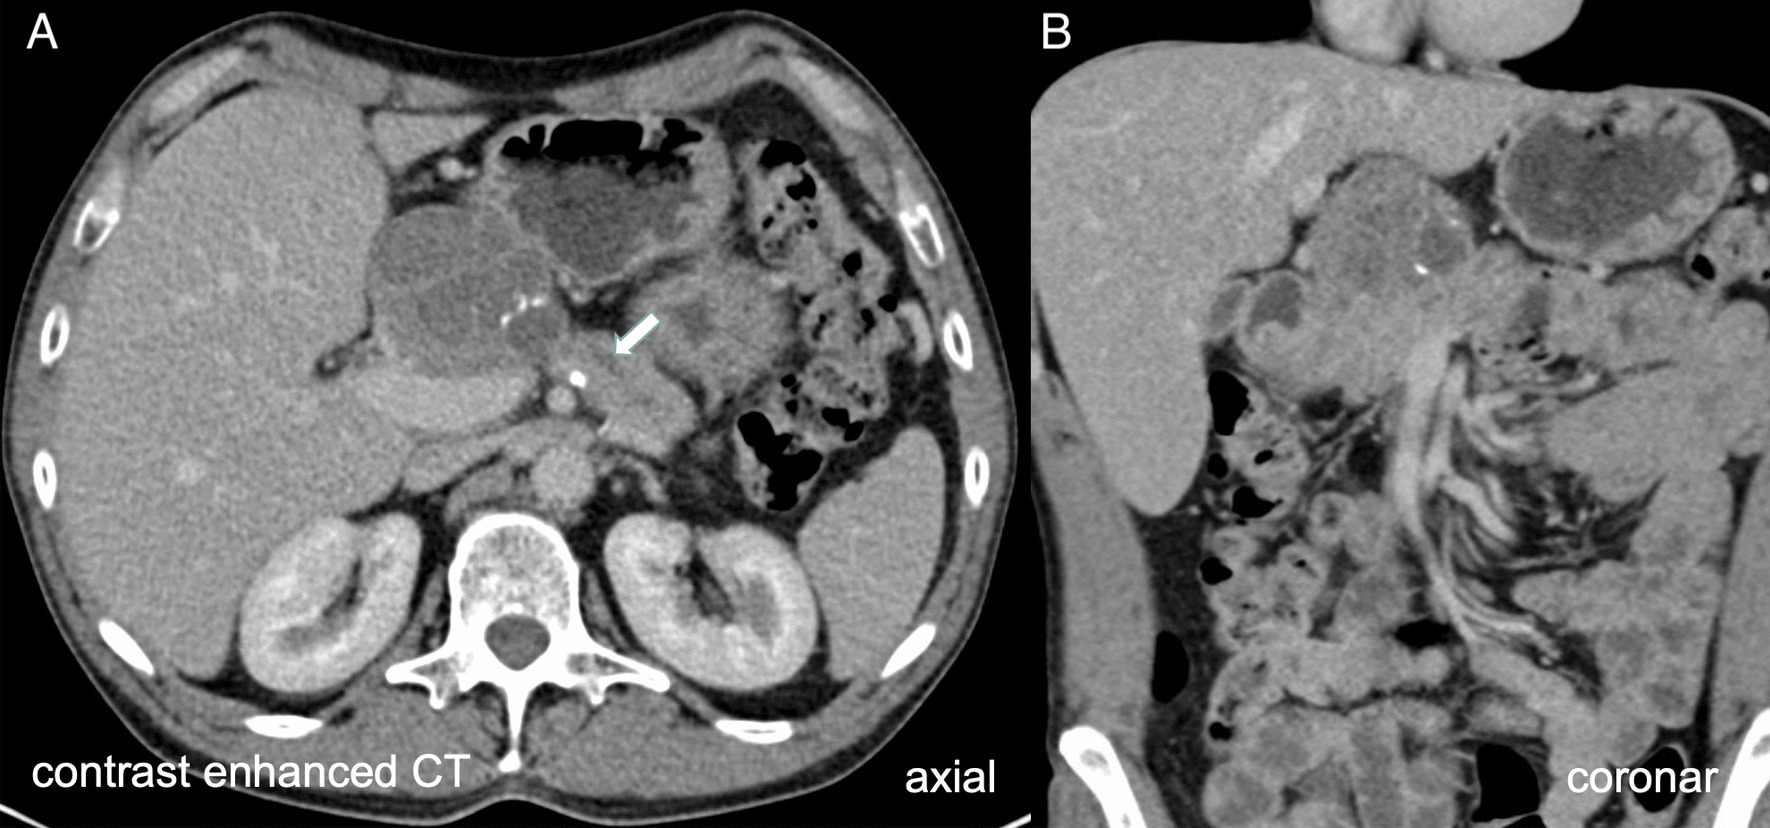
Contrast enhanced computed tomography (CT) scan revealed a septated cystic lesion with solitary calcification in axial (A) and coronar (B) view with potential infiltration of the duodenum. The pancreatic duct was shown dilated up to 6mm (arrow). The dignity of the lesion cannot be conclusively clarified
